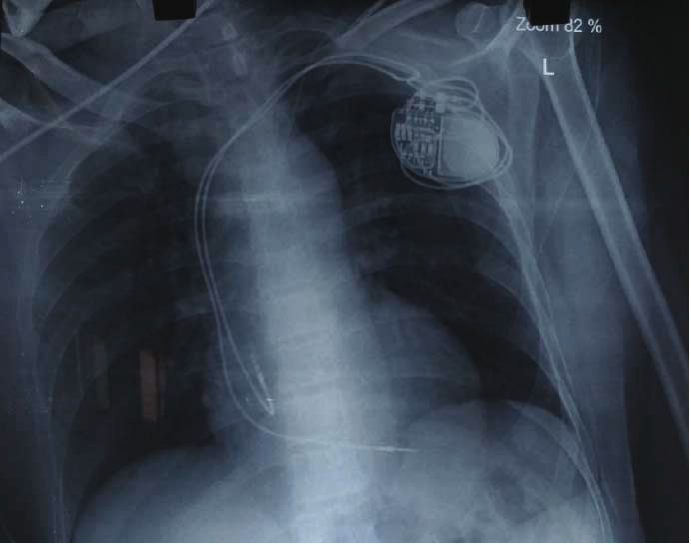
Chest X-ray showing dual-chamber permanent pacemaker (pulse generator with leads in right atrium and right ventricle).
